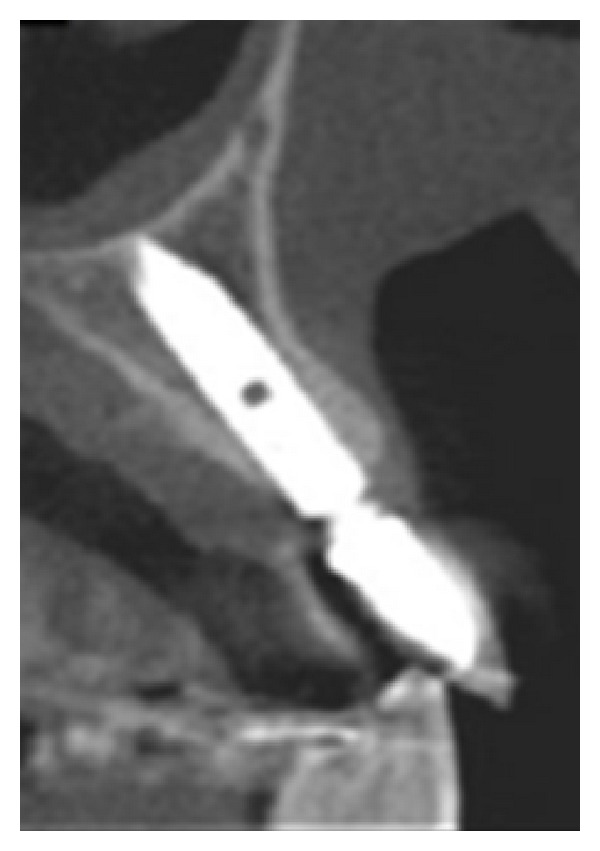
Final computed tomography.
